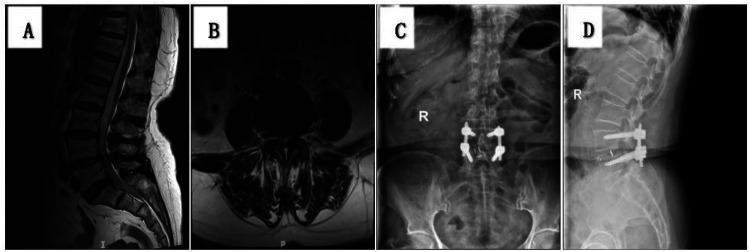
(A,B) preoperative MRI showing L4/5 lumbar disc herniation; (C,D) lumbar anteroposterior and lateral reexamination 3 days after surgery showing good position of internal fixation.
